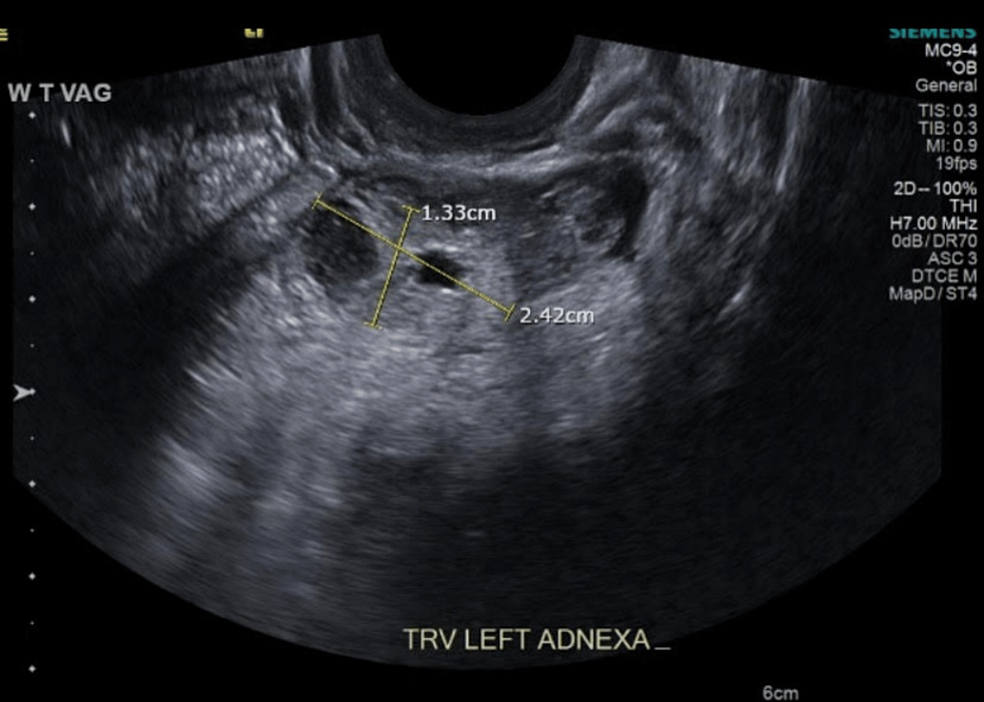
Transvaginal ultrasonogram. The sagittal image shows the complex area measuring 24 × 13 mm in the left ovary.
